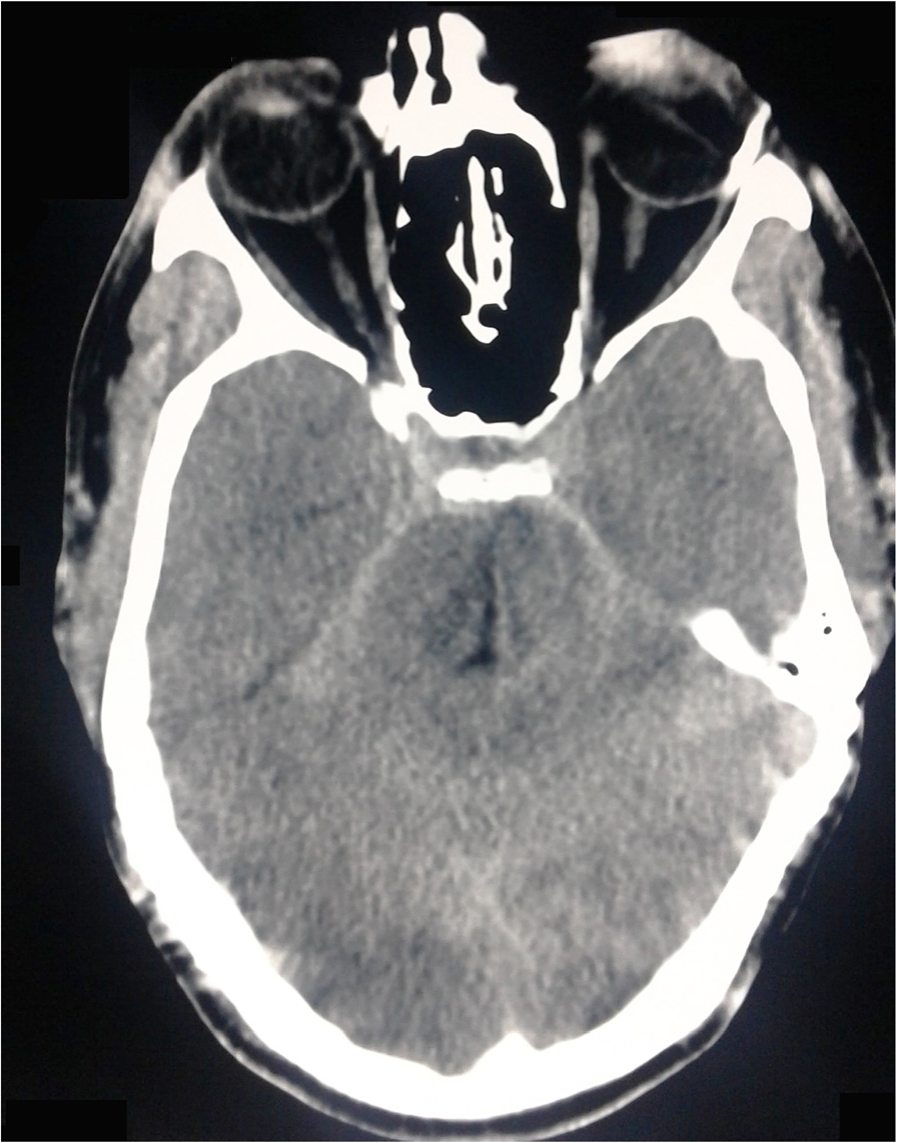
Computed tomography image of the head; the axial section of the brain is shown. The image shows linear hyperdense areas in the ambient and suprasellar cisterns suggestive of subarachnoid bleeding
